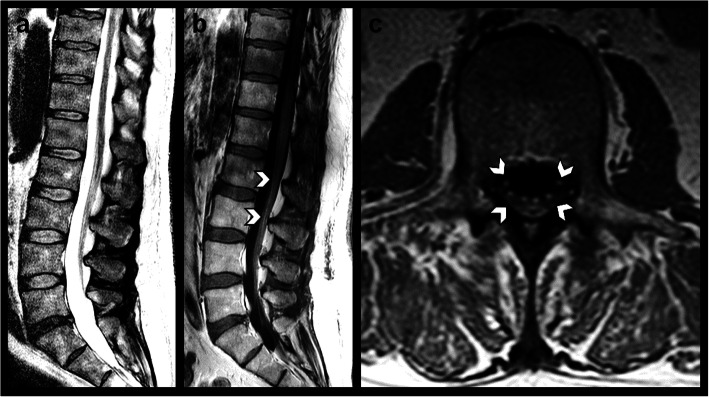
Contrast-enhanced whole-spine MRI performed 2 h after symptom onset. a Sagittal T2-weighted image showing no signal abnormalities of the spinal cord. b Sagittal and c axial T1-weighted images after administration of gadolinium-based contrast agent revealing smooth contrast enhancement of the conus medullaris and the anterior and posterior roots of the cauda equina (arrows) extending beyond the spinal canal into the lumbosacral nerve roots. All MR imaging was performed on a clinical 1.5 T MR scanner (Achieva, Philips Healthcare, Best, the Netherlands) and included a T1-weighted spin-echo sequence (405/7 ms [repetition time (TR)/echo time (TE)] before and after administration of a Gd-DO3A-butrol-based contrast agent (Gadovist, Bayer, Leverkusen, Germany) in axial and sagittal orientation, a T2-weighted turbo spin-echo sequence [2800/90 ms (TR/TE)] as well as a sagittal T2-weighted modified Dixon (mDixon) spin-echo sequence [2800/90 ms (TR/TE)]
